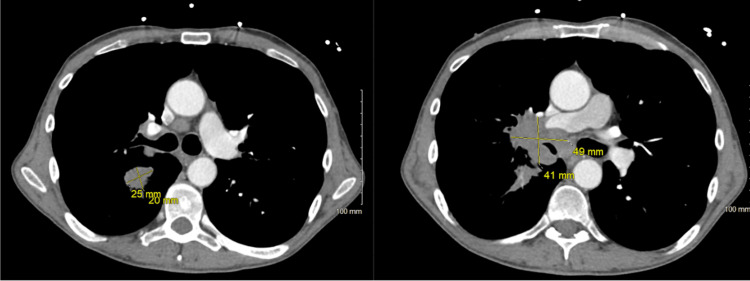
Chest CT revealing two images at two separate planes showing two different right-sided lung masses.
